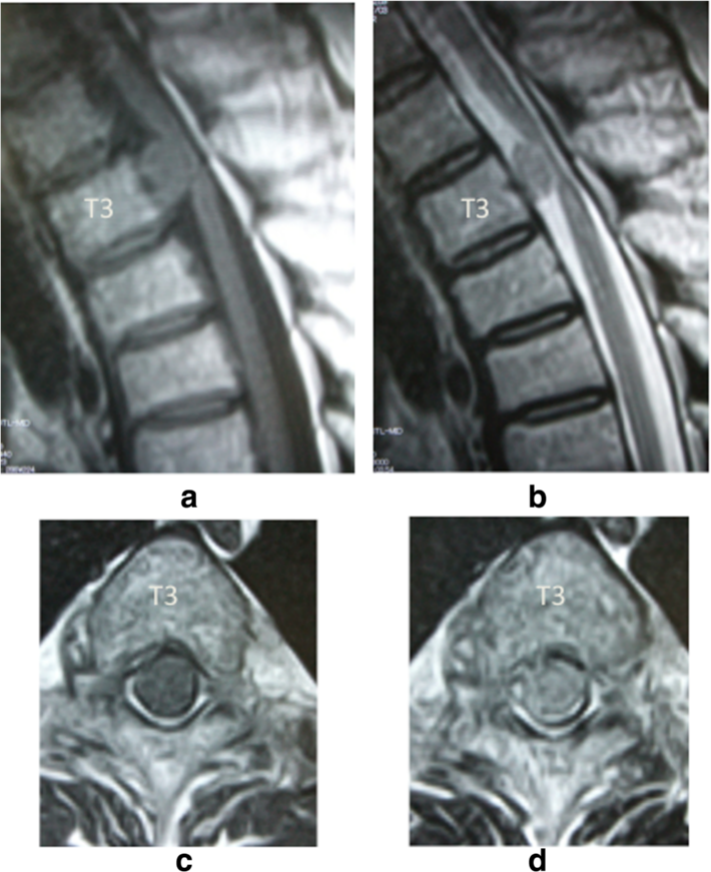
Preoperative magnetic resonance images. Sagittal T1-weighted (a) and T2-weighted (b) magnetic resonance images of the thoracic spine, demonstrating an intradural extramedullary mass anterior to the spinal cord at the T3 level. The mass was iso-intense on T1-weighted imaging and T2-weighted imaging. Axial T1-weighted (c) and T2-weighted (d) magnetic resonance images show that the tumor seemed to be completely covered by the spinal cord.
